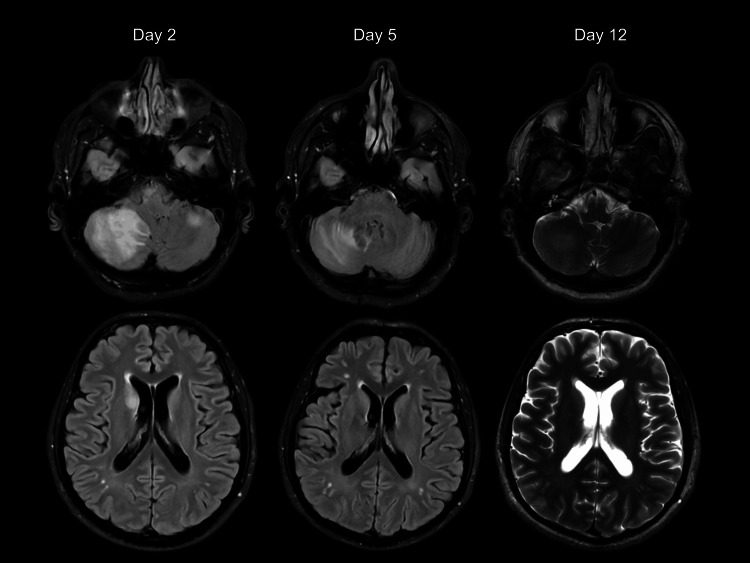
Serial MRI head demonstrating interval resolution of multifocal intracranial lesions Serial axial MRI head (T2-weighted and FLAIR sequences on days 2 and 5 and contrast-enhanced T2-weighted sequences on day 12) demonstrates progressive interval resolution of multifocal intracranial lesions. Initial non-contrast imaging shows a right cerebellar lesion with vasogenic edema and additional multifocal involvement. Follow-up imaging demonstrates gradual resolution, with improvement by day 12. FLAIR: fluid attenuated inversion recovery
